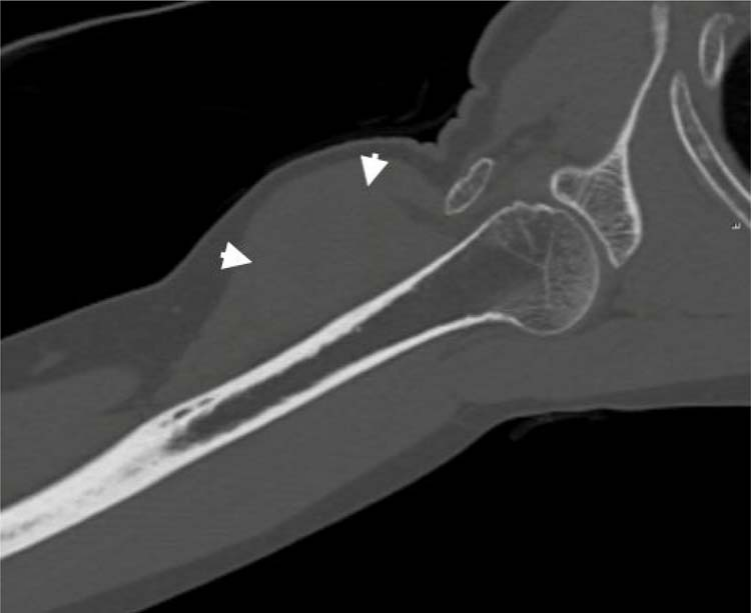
Coronal CT of the right arm. Short arrows indicate the tumoral mass.
